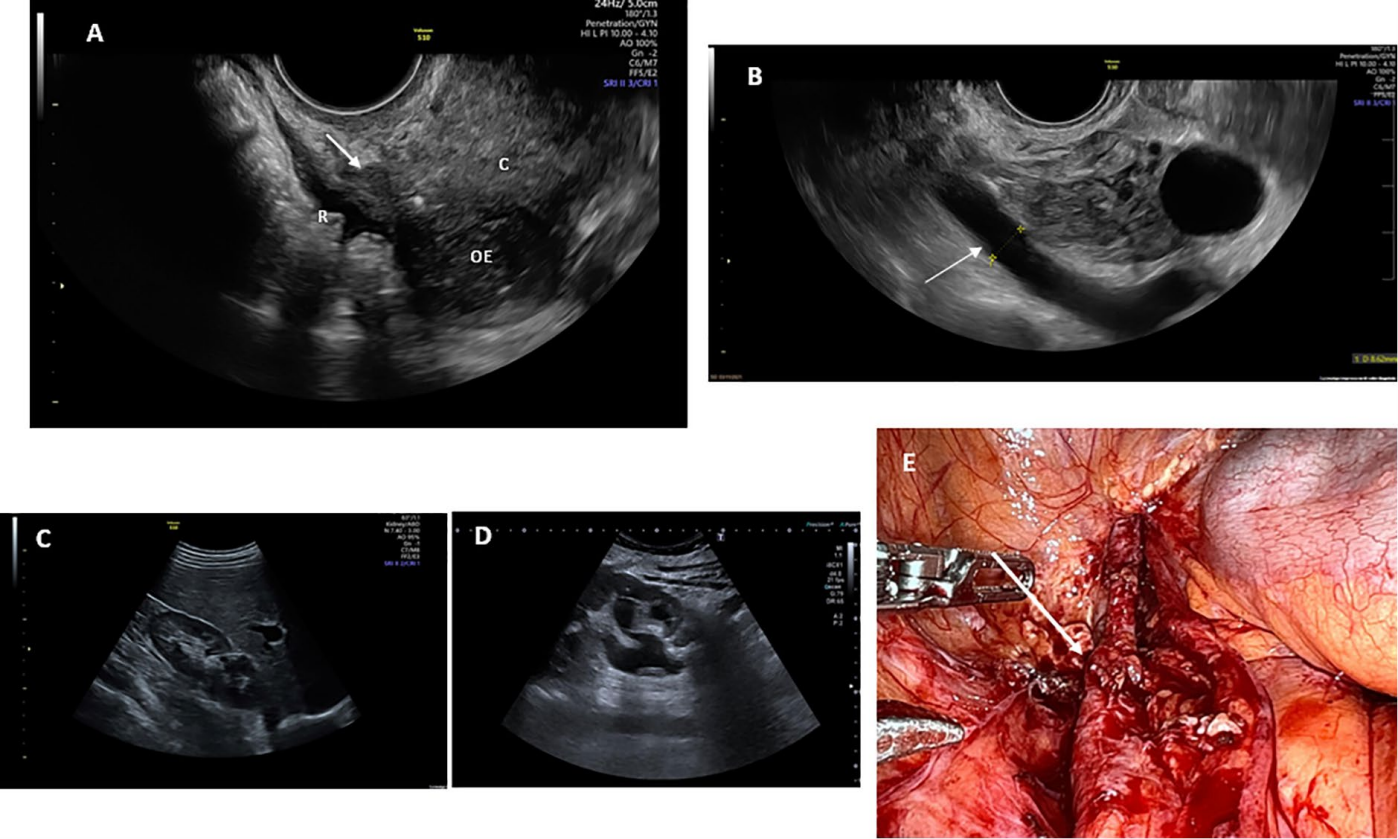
(A) Arrow: Sonographic image of hypoechoic thickening of the uterosacral ligament; C: Cervix; R: Rectum; OE: Ovarian Endometrioma. (B) Arrow: Sonographic image of ureteral dilatation by the left adnexal space. (C) Normal kidney. (D) Hydronephrosis secondary to distal ureteral stenosis due to deep endometriosis nodule. (E) Surgical image of distal ureteral stenosis (arrow) and upper dilatation due to deep endometriosis pelvic nodule.
